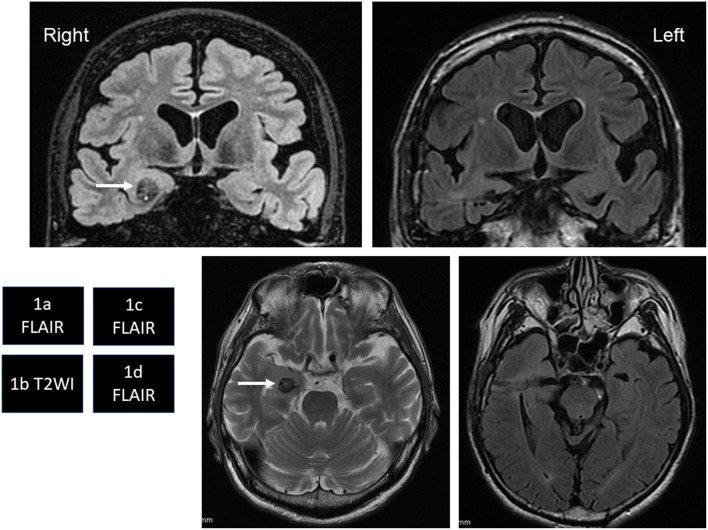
(a,b) White arrows in coronal fluid-attenuated inversion recovery (FLAIR) image (a) and axial T2-weighted image (b) show right temporal cavernous malformation (CM). Cortical atrophy and ventriculomegaly of the brain are also seen. (c,d) FLAIR images show total removal of the CM by a transcortical approach.
